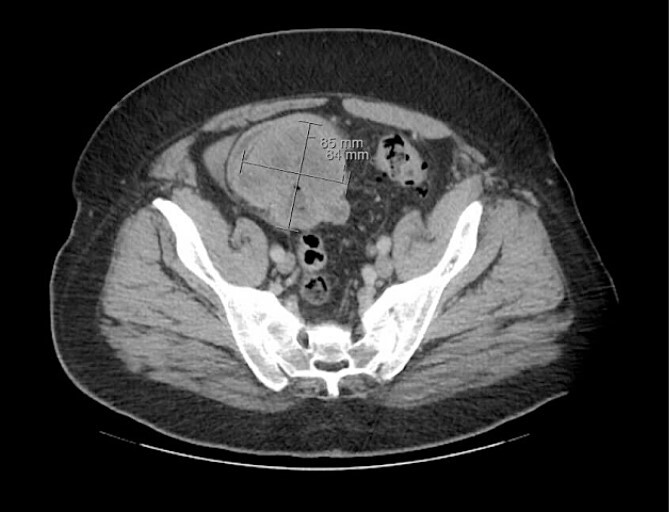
Initial CT imaging, axial cut, showing necrotic mass causing small bowel obstruction.
